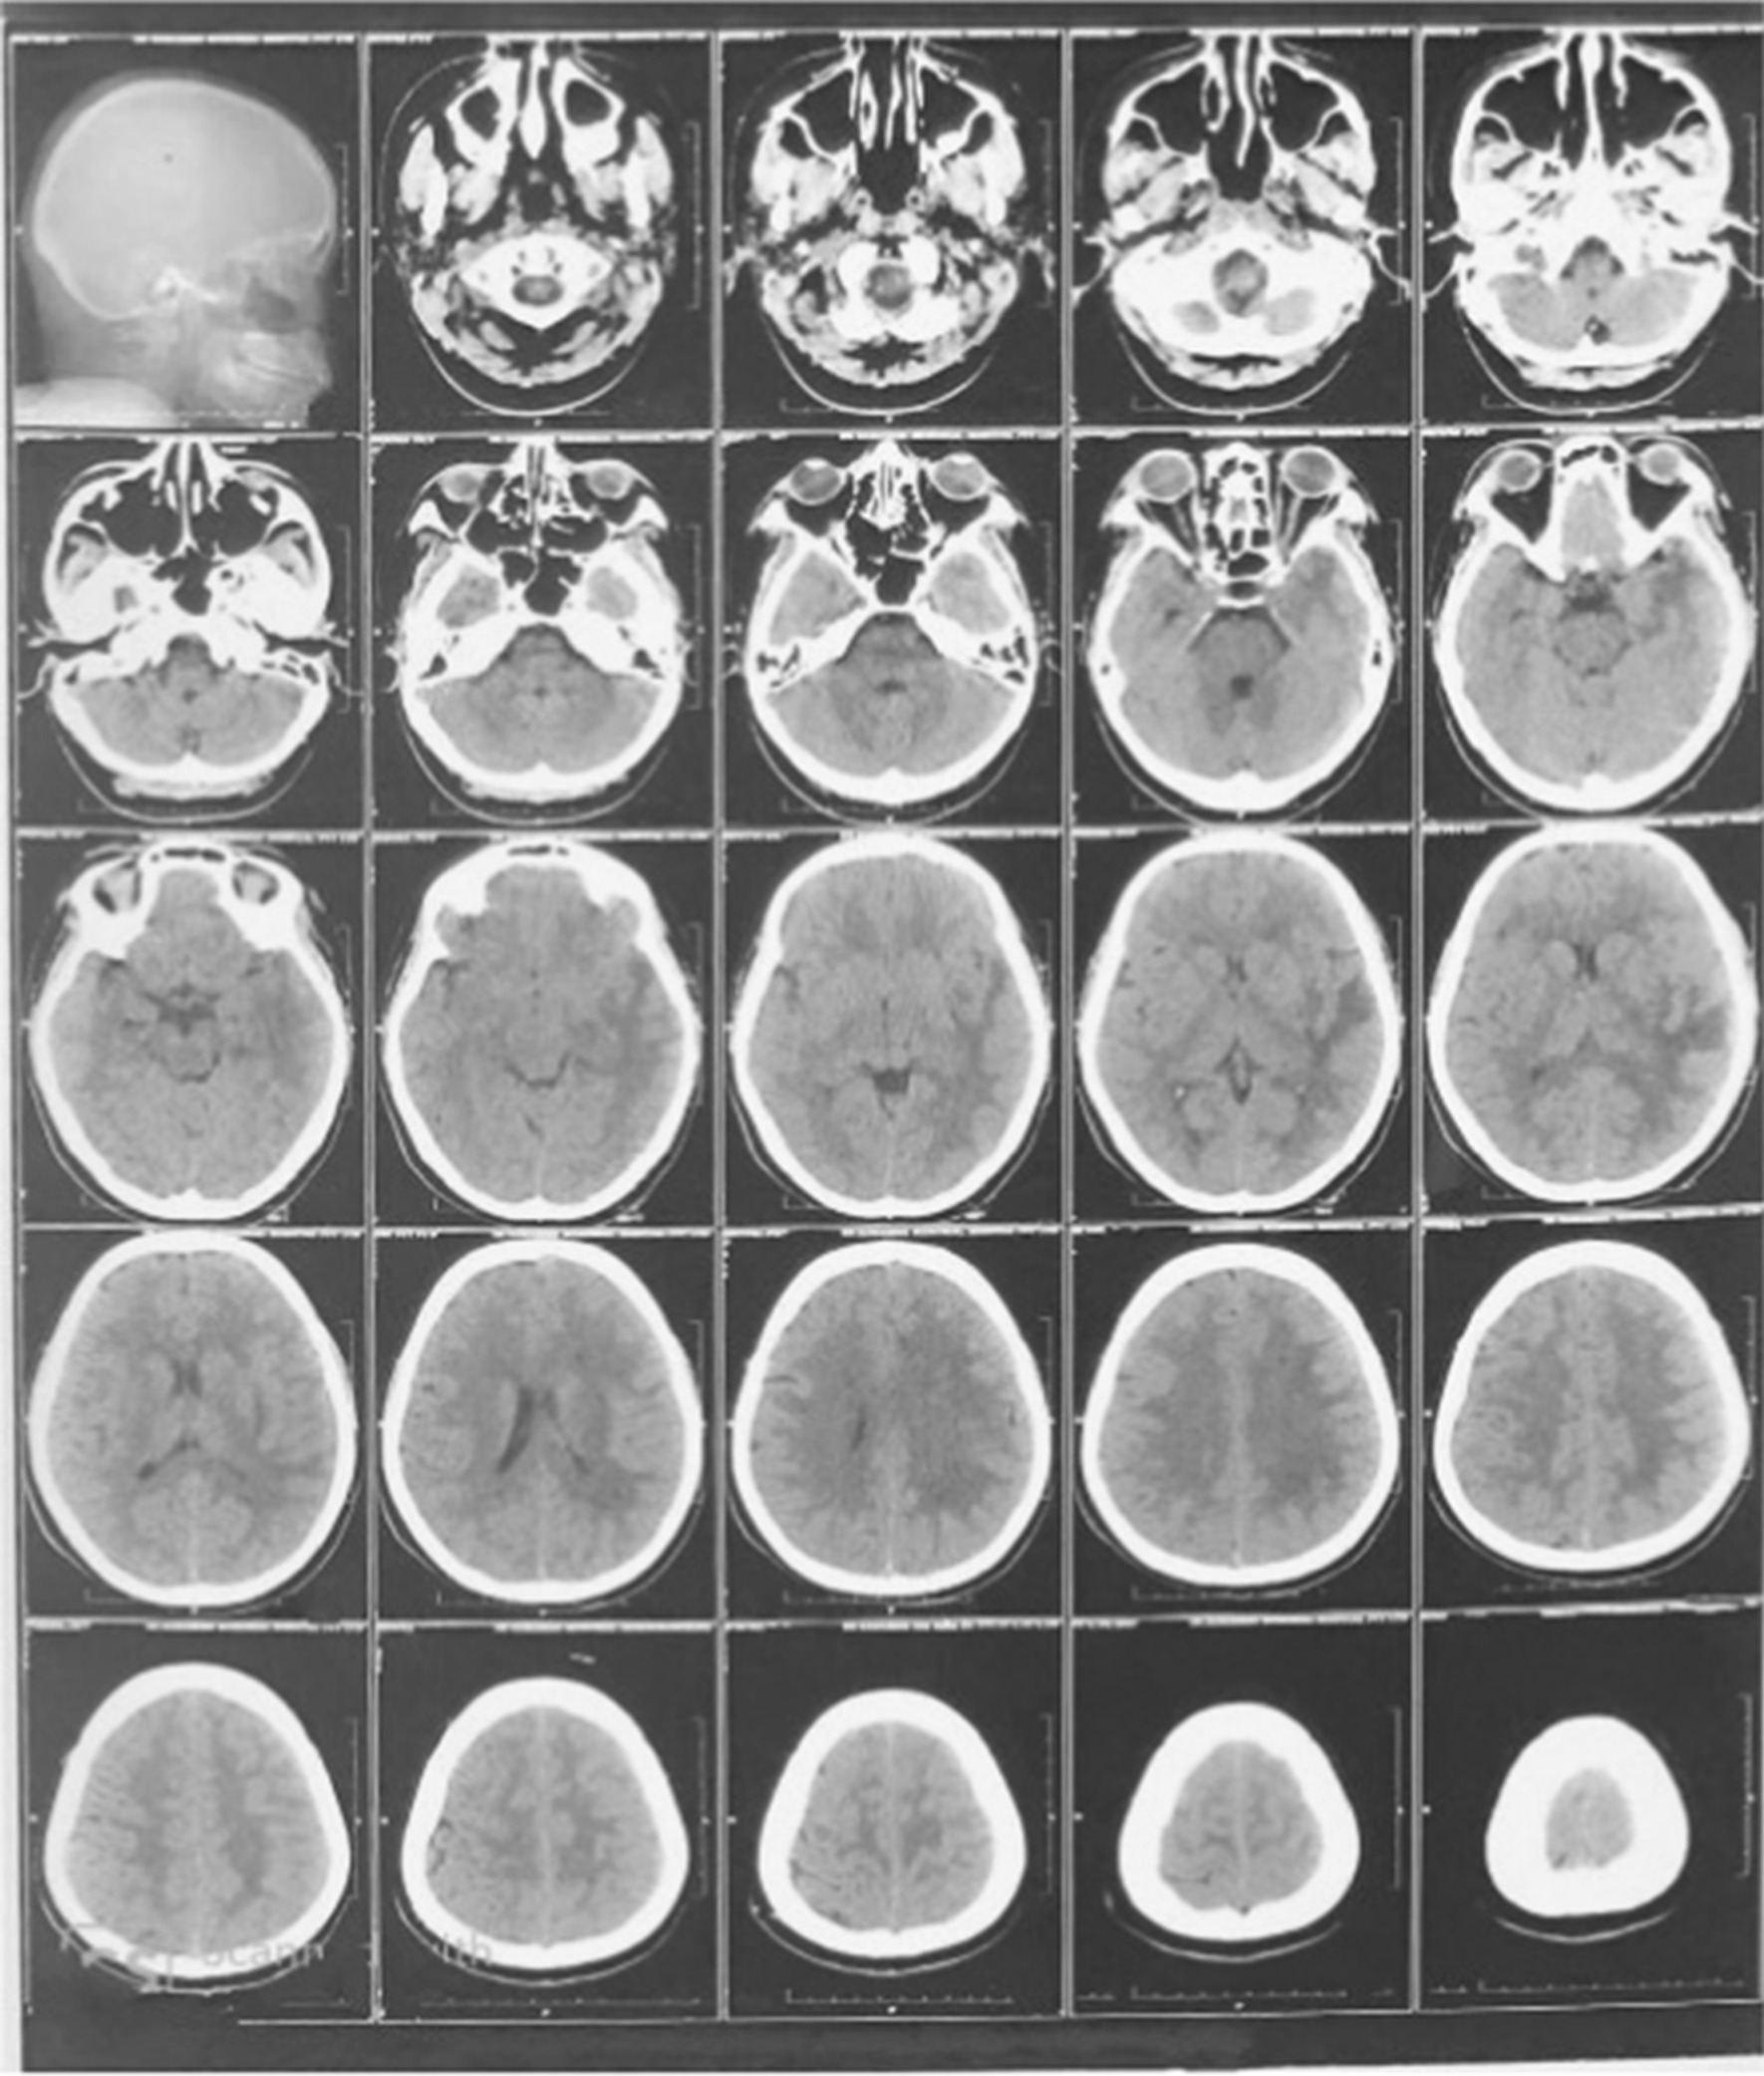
Computed Tomography (CT) scan. Brain showing ill-defined areas of parenchymal Edema in left parieto temporal lobe
